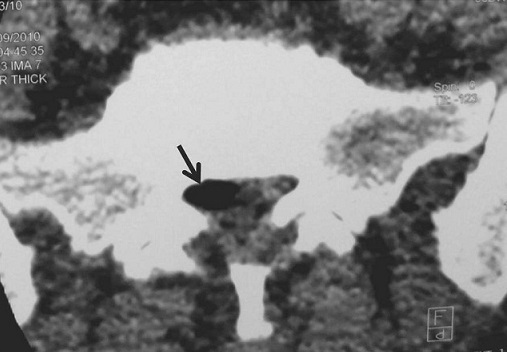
Computed tomography (CT) axial view showed a 10 mm gas bubble (arrow) in the right anterolateral portion of the epidural space at L5-S1 level compressing the dural sac and right S1 nerve root
